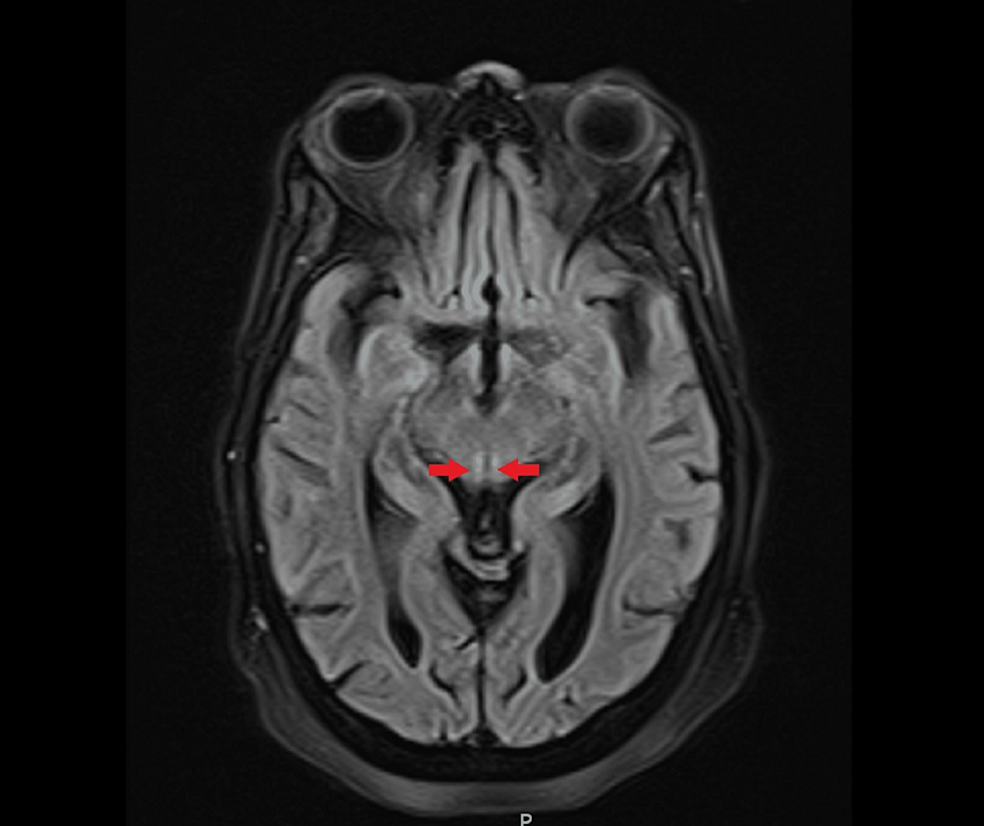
On magnetic resonance imaging (MRI) of the brain, the red arrows show a symmetric hypertense signal within the periaqueductal gray matter.
